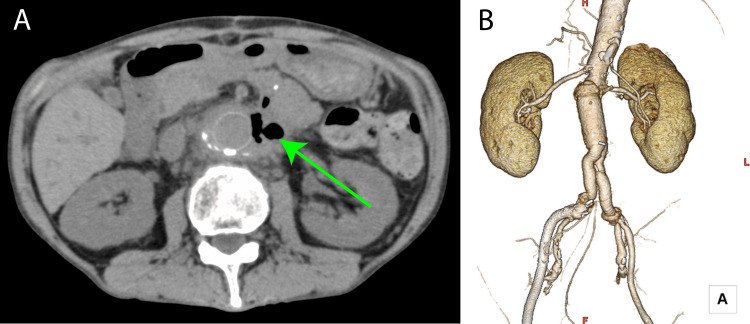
Preoperative enhanced computed tomography (A) Horizontal section image. The air around the artificial vessel was observed. (green arrow); (B) Volume rendering image. The abdominal aorta had been replaced by an artificial vessel by the previous physician.
